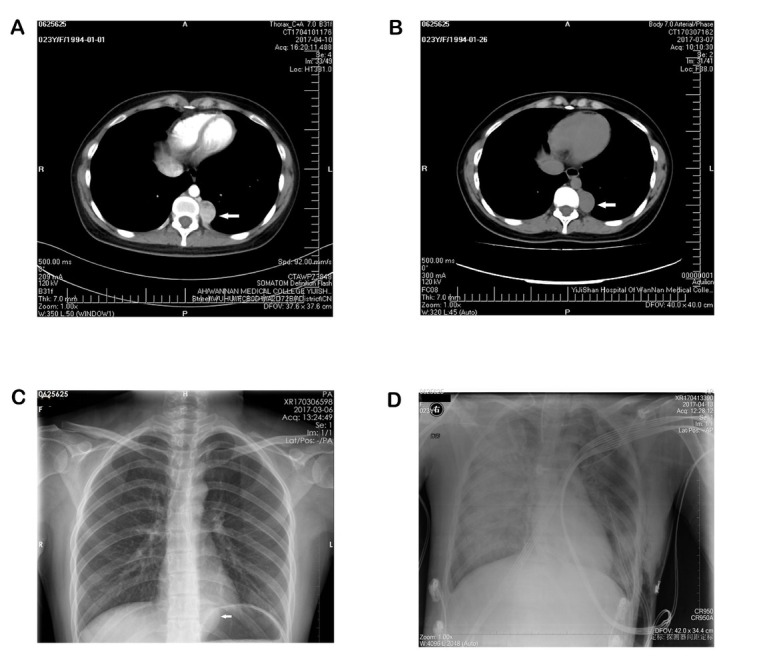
Radiologic examination. (A/B) Thoracic contrast-enhanced CT showing a 2.5x2 cm mass at left posterior mediastinum (white arrow) with heterogeneous density. (C) Posteroanterior chest x-ray showing a left posterior mediastinum soft-tissue mass (white arrow). (D) Chest X-ray 12 hours after operation showing severe interstitial and alveolar oedema.
